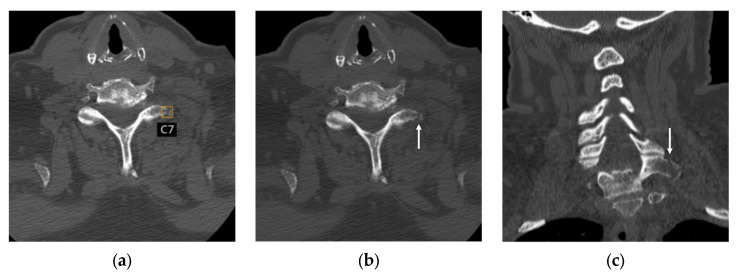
Non-enhanced CT images in a 69-year-old male with an acute fracture of the left transverse process of C7. The first radiologist classified the case as “negative”, whereas the second and third radiologists identified the fracture, representing a discrepant case between the first two radiologists. (a) The AI application correctly localized the fracture using a bounding box and accurately labeled the spinal level. (b,c) Corresponding axial (b) and coronal (c) CT images without bounding boxes show the fracture of the left C7 transverse process (arrows).
